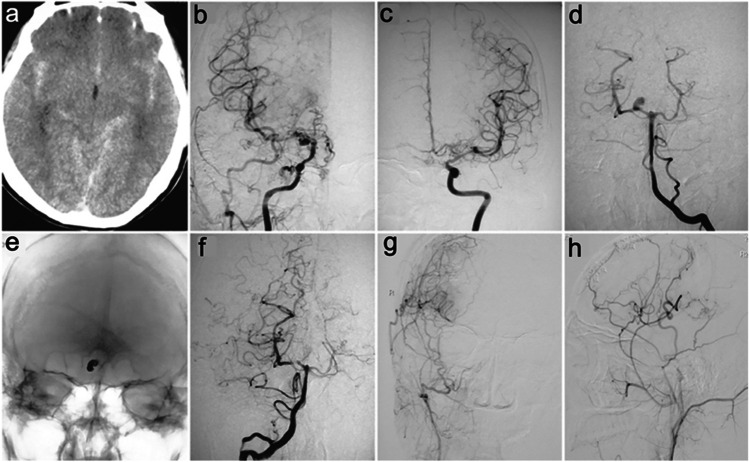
A 40-year-old MMD patient with severe explosive headache was found to have a saccular aneurysm by DSA. The aneurysm was occluded by coil embolization immediately after DSA. Right STA-MCA bypass combined with EDMS was performed 1 month after SAH ictus. a CT scan revealed SAH in the longitudinal fissure, Sylvian fissure, and quadrigeminal cistern. b and c Bilateral ICA angiography studies showing MMD with Suzuki Stage III. d Vertebral angiography study showing a saccular aneurysm at the P1/P2 junction of the right PCA. e and f Follow-up vertebral angiography studies showing complete obliteration of the aneurysm. g and h ECA angiography studies showing Matsushima Grade A collateral compensation supplied by the anastomosis [50]
